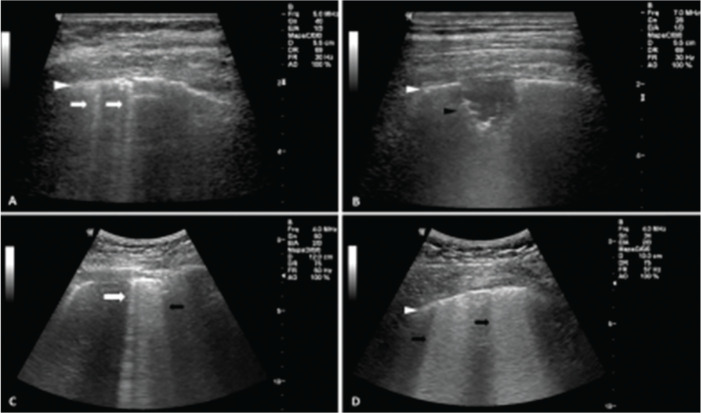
Lung ultrasound. Linear (A and B) and convex (C and D) transducers in intercostal spaces show irregular and thickened pleural line (white arrowhead); hypoechogenic image with irregular contours, compatible with subpleural consolidation (black arrowhead); multifocal B-lines (white arrow), some of which are coalescent (black arrow), characterizing the appearance of a white lung on ultrasound (A).
